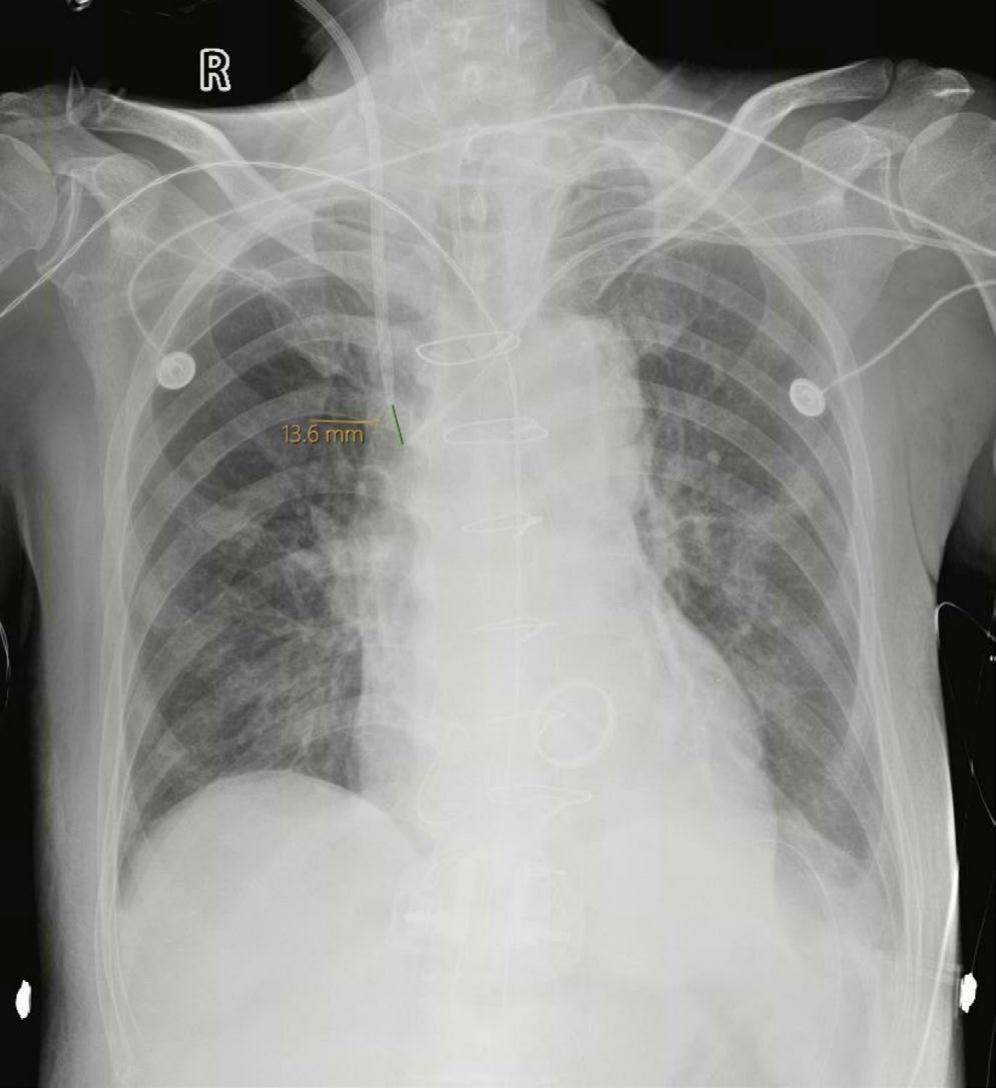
Chest radiograph showing the positions of the central venous and dialysis catheters.
